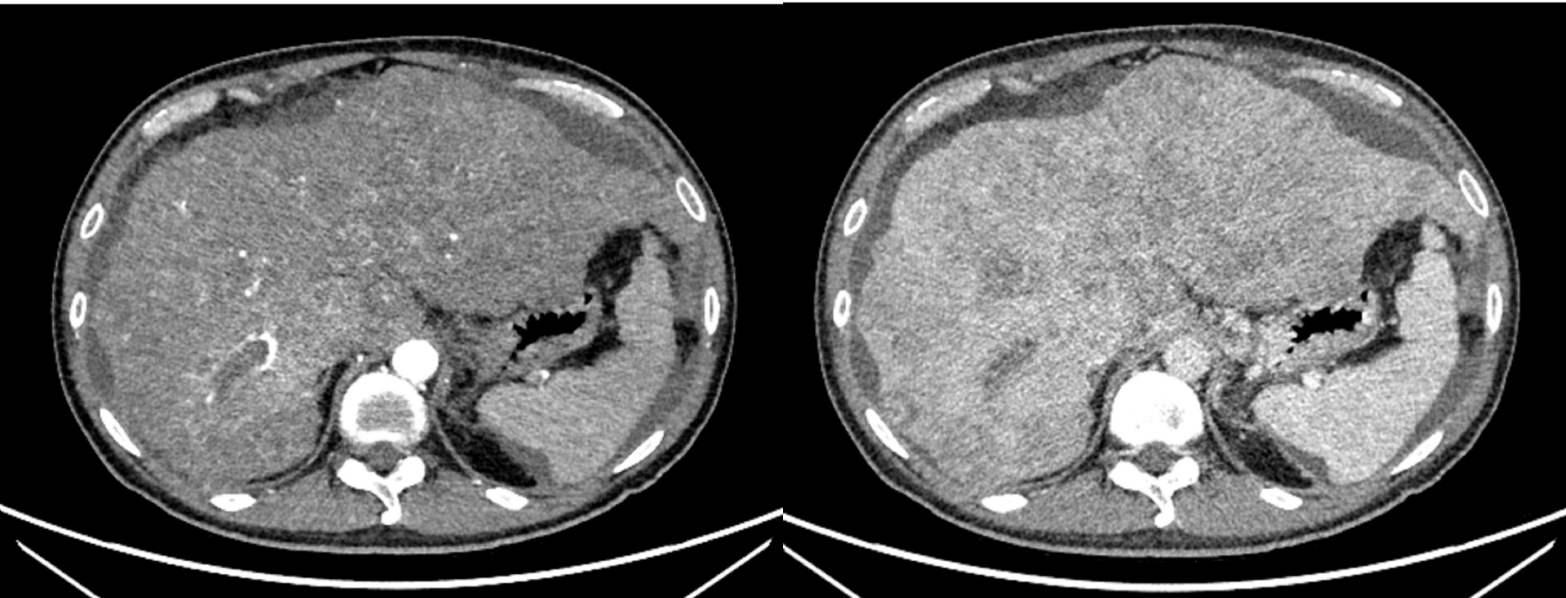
The preoperative CT images of the liver.
